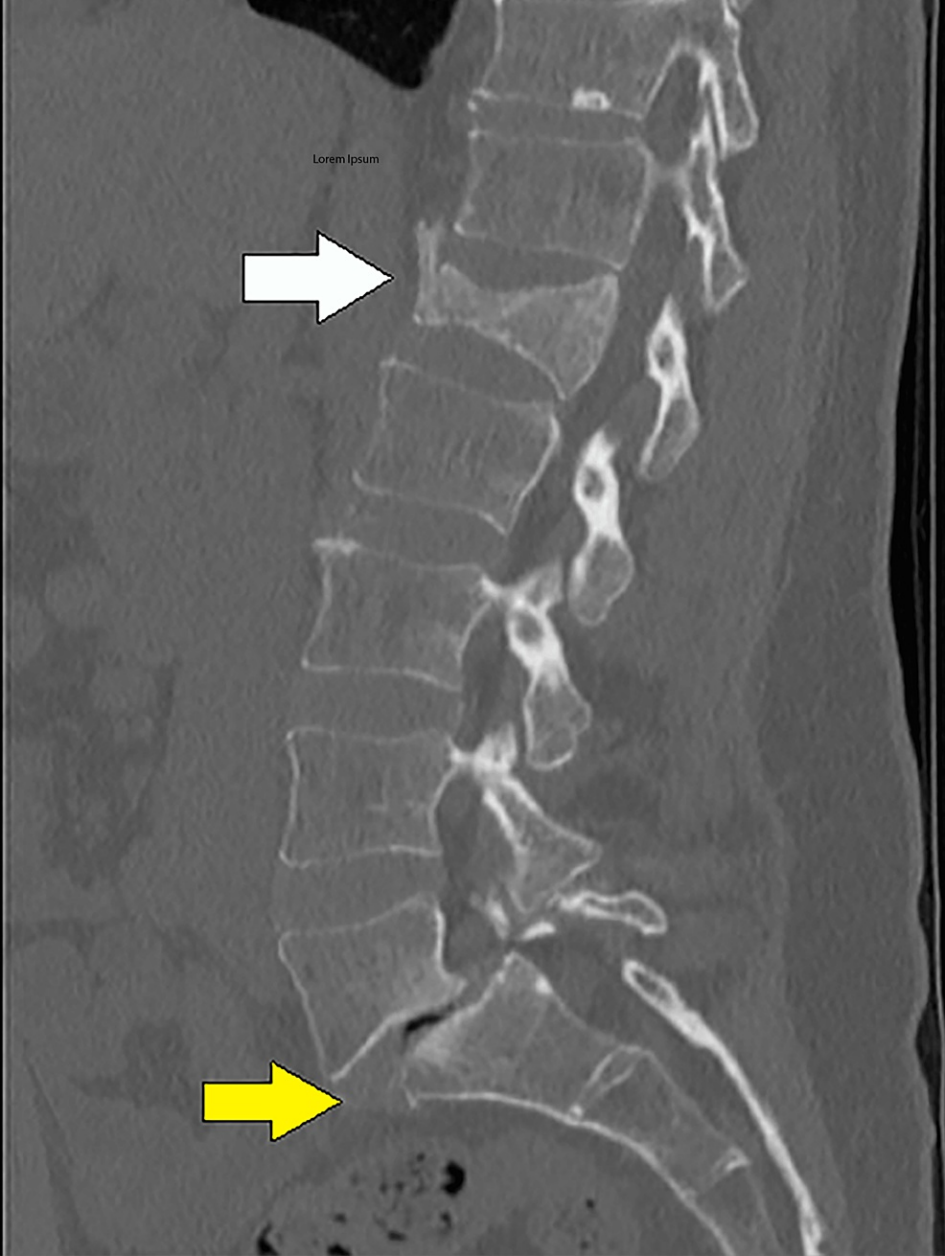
CT scan of the lumbar spine White arrow: L1 compression fracture; Yellow arrow: L5 pars defect
